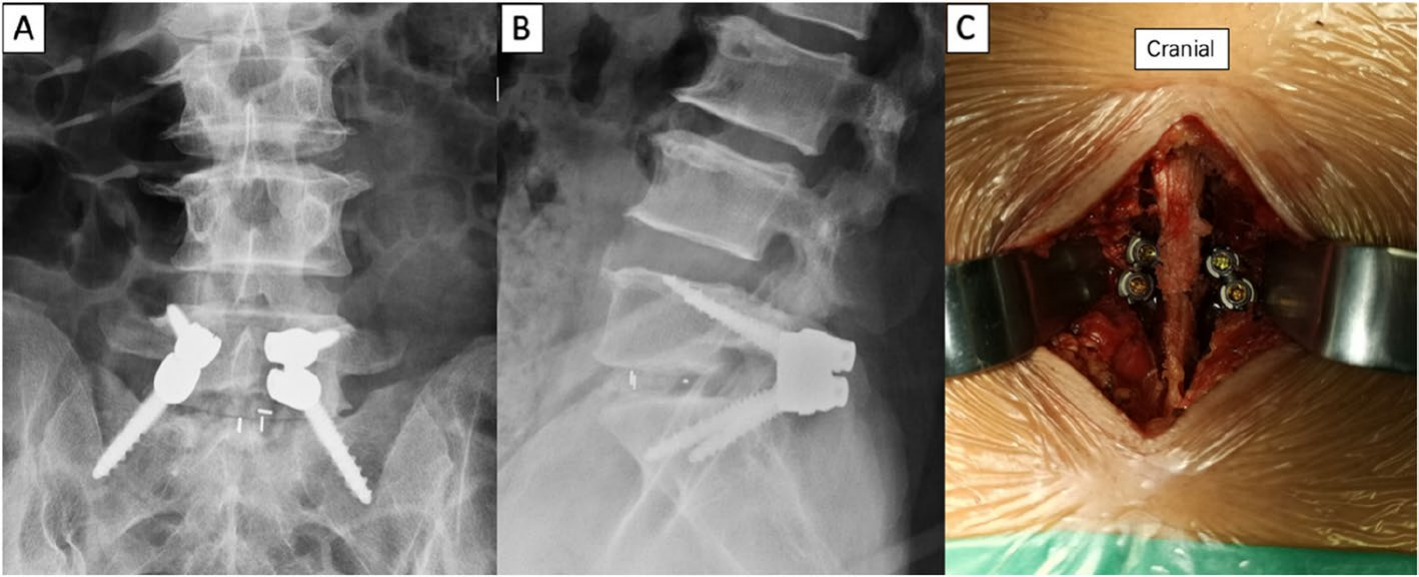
A LP-PLSIF postoperative coronal lumbar spine X-ray. B Postoperative sagittal lumbar spine X-ray. C LP-PLSIF intraoperative picture
